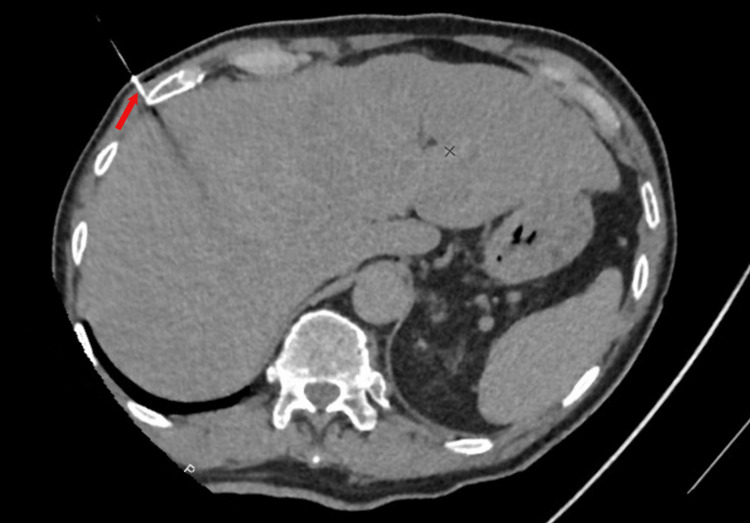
Abdominal CT scan performed to guide the hepatic nodule biopsy. The arrow shows the inserted biopsy needle.
